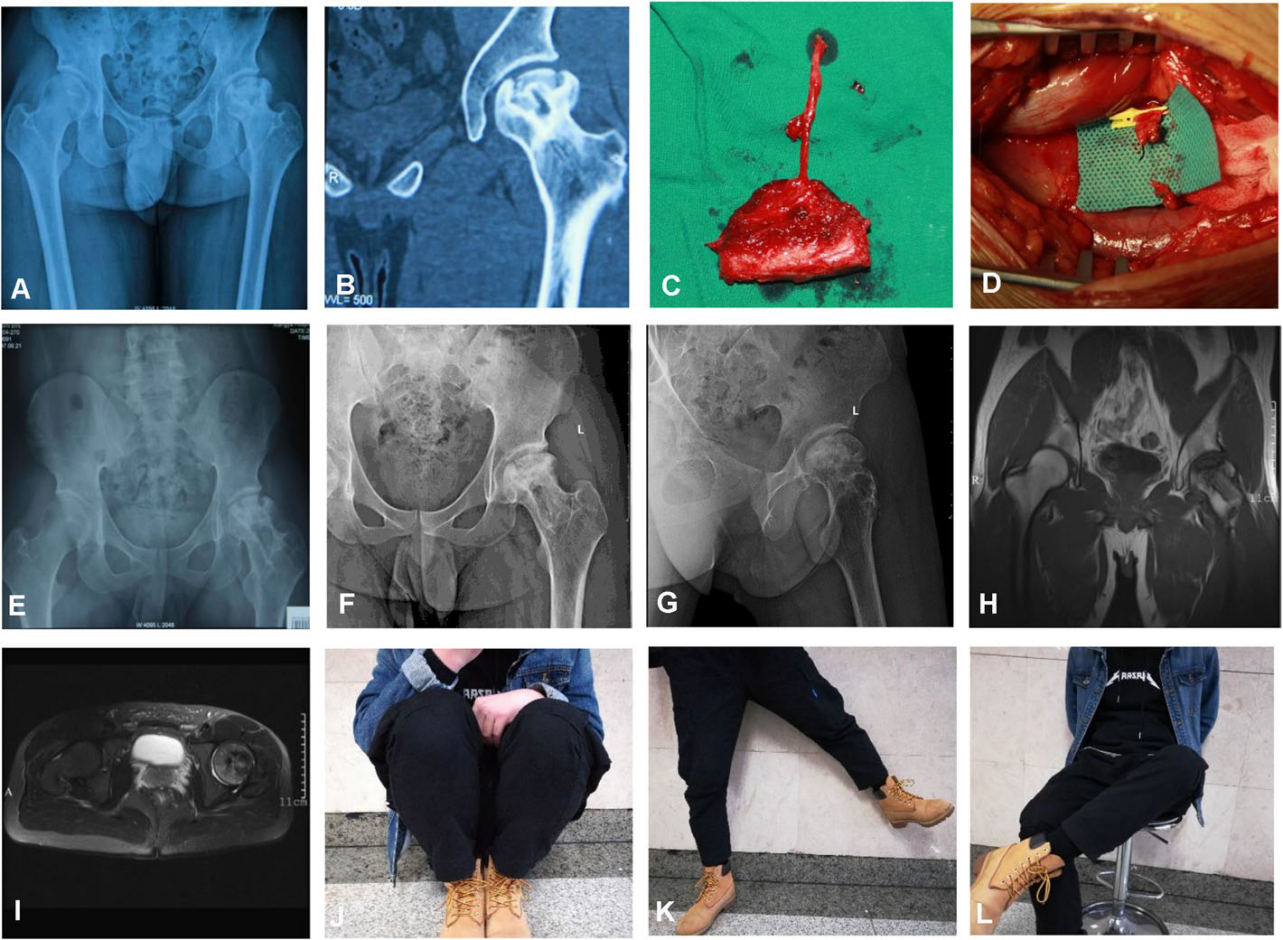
A 19-year-old male patient underwent free graft of vascularized iliac bone flap based on deep circumflex iliac vessels for treatment of traumatic ONFH of the left side. a Preoperative X-ray and b preoperative CT scans showed heterogeneous density of the femoral head. c The iliac bone flap was separated. d The cancellous bone was implanted into the femoral head, and the iliac bone flap was inserted into the bone groove. Postoperative anteroposterior (AP) X-rays at e 9 months and f 27 months, lateral X-rays at g 27 months and postoperative MRI scans of h AP view and i lateral view showed no collapse of femoral heads or narrowing of the hip joint spaces. Hip j flexion, k abduction, and l external rotation showed good recovery
